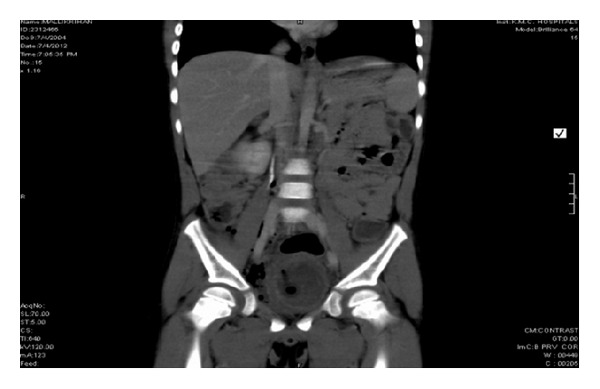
Contrast CT showing diffuse thickening of the right posterolateral bladder wall.
